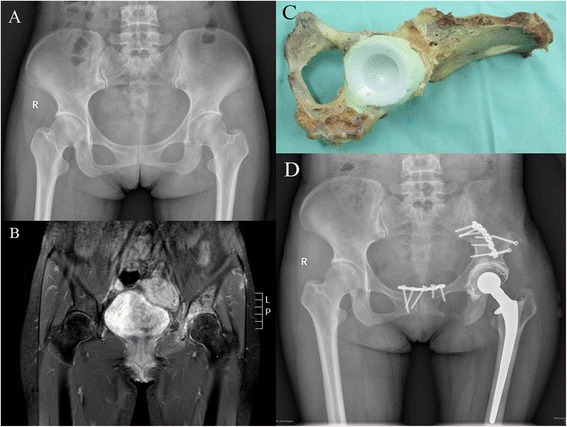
Patient 7 was a 48-year-old female with chondrosarcoma. a Preoperative AP radiograph. b Preoperative MRI. c Pasteurized autograft ready to be returned. d AP radiograph 54 months postoperatively. Bone resorption was observed at the inferior ramus of the pubis
